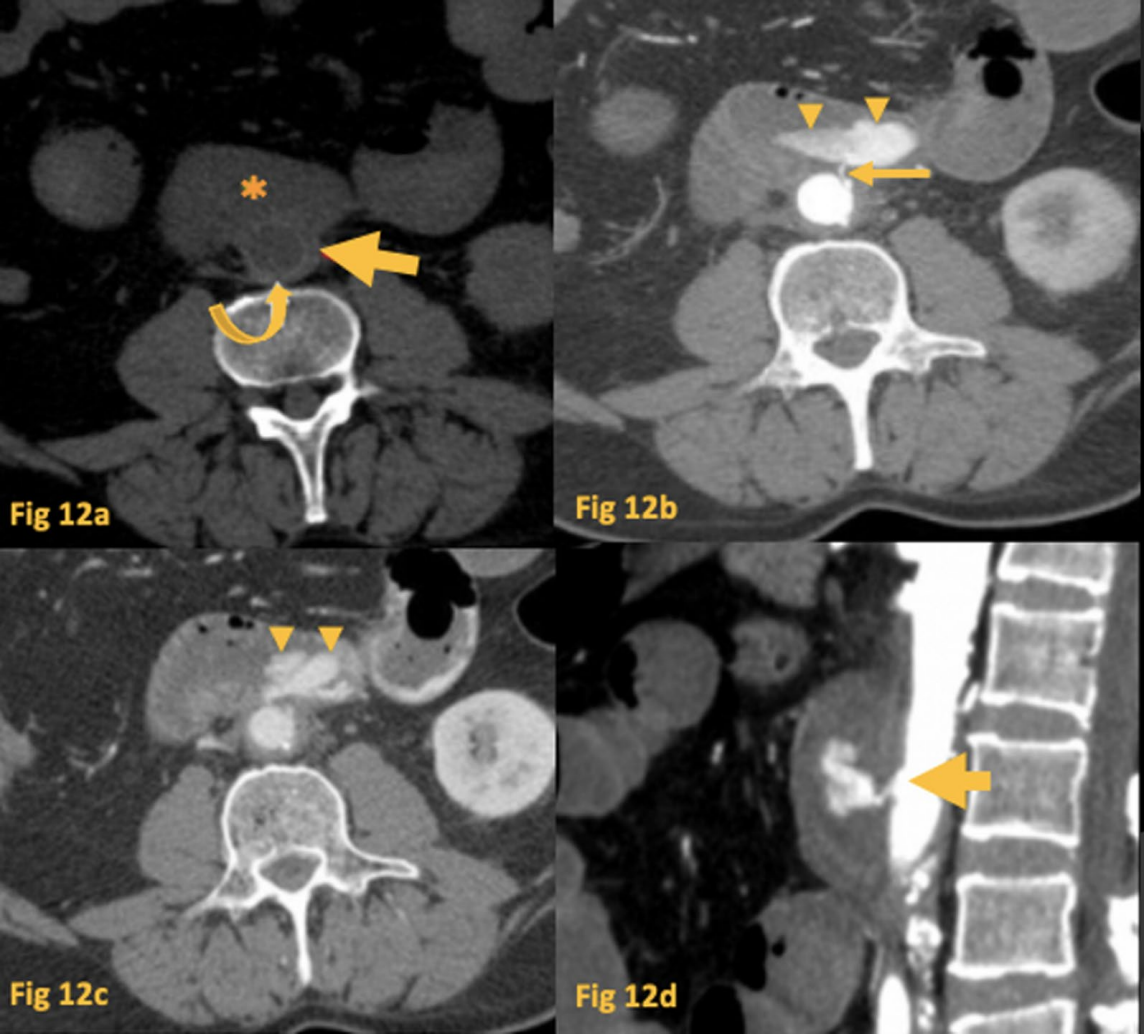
Fistula (Aorto-duodenal). Unenhanced CT (a) of patient with hematemesis shows an aorto-bi-femoral stent (curved arrow) inside the infra-renal aorta, with exclusion of atherosclerotic plaque (arrow), in close proximity to the third part of the duodenum (asterisk). Arterial phase CT (MIP) (b) shows an aortoduodenal fistula (arrow), with blood leaking into D3 (arrowheads) in the topography of the proximal end of the aortic stent. Portal phase CT (c) shows increased pooling of blood in the duodenum and jejunum (arrowheads). Sagittal reformat in arterial phase CT (d) better shows the fistulous tract of the blood into D3 (arrow)
